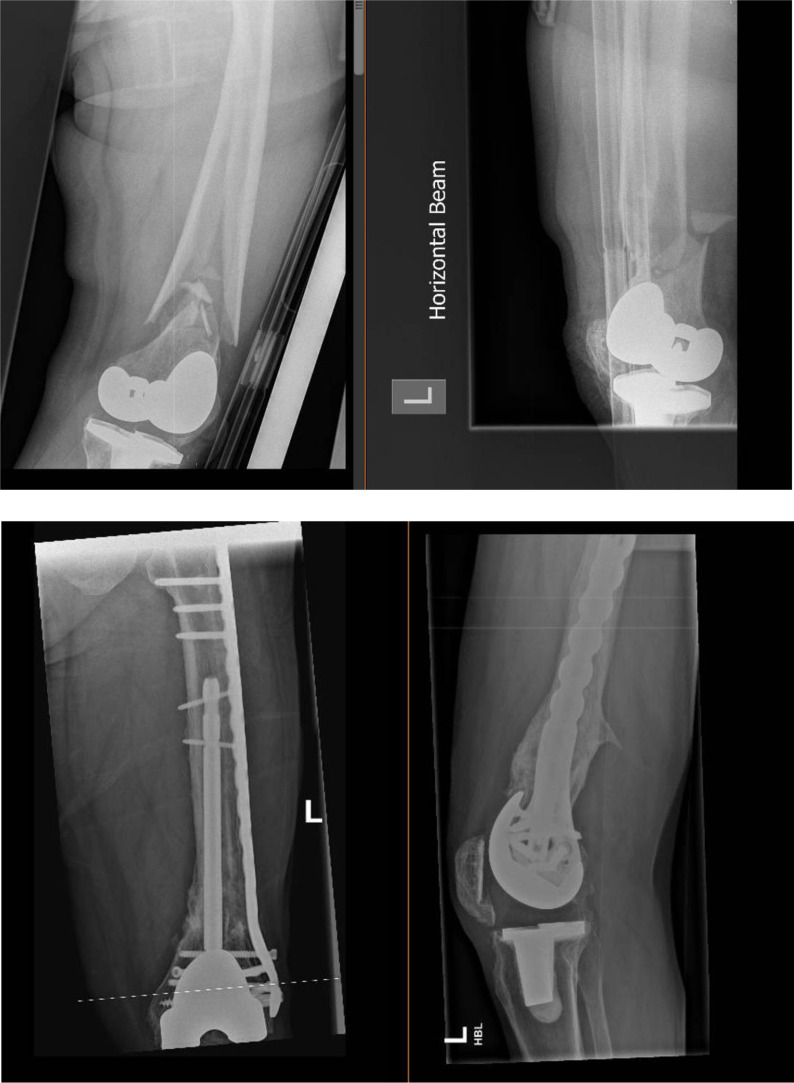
Pre-operative and post-operative radiographs (fracture union) of linked nail/plate construct of distal femur periprosthetic fracture (combination of short rIMN/long bridging LP).
